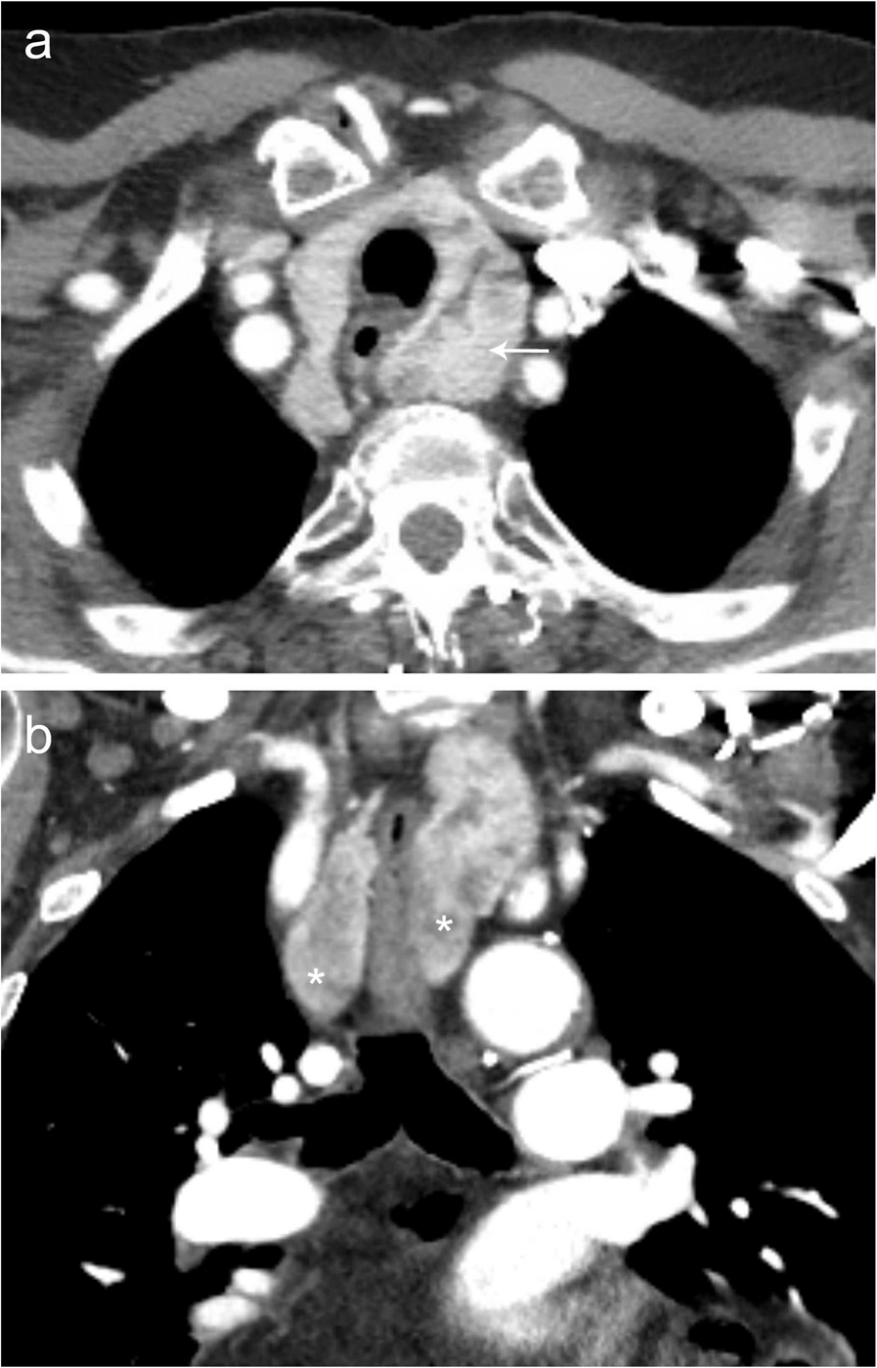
Multinodular thyroid goiter. Axial (a) and coronal (b) contrasted CT imaging demonstrates an enlarged heterogeneous multinodular appearance of the thyroid gland. Note that this enlargement is causing rightward deviation of the trachea (arrow) and substernal extension (asterisk) into the anterior mediastinum
